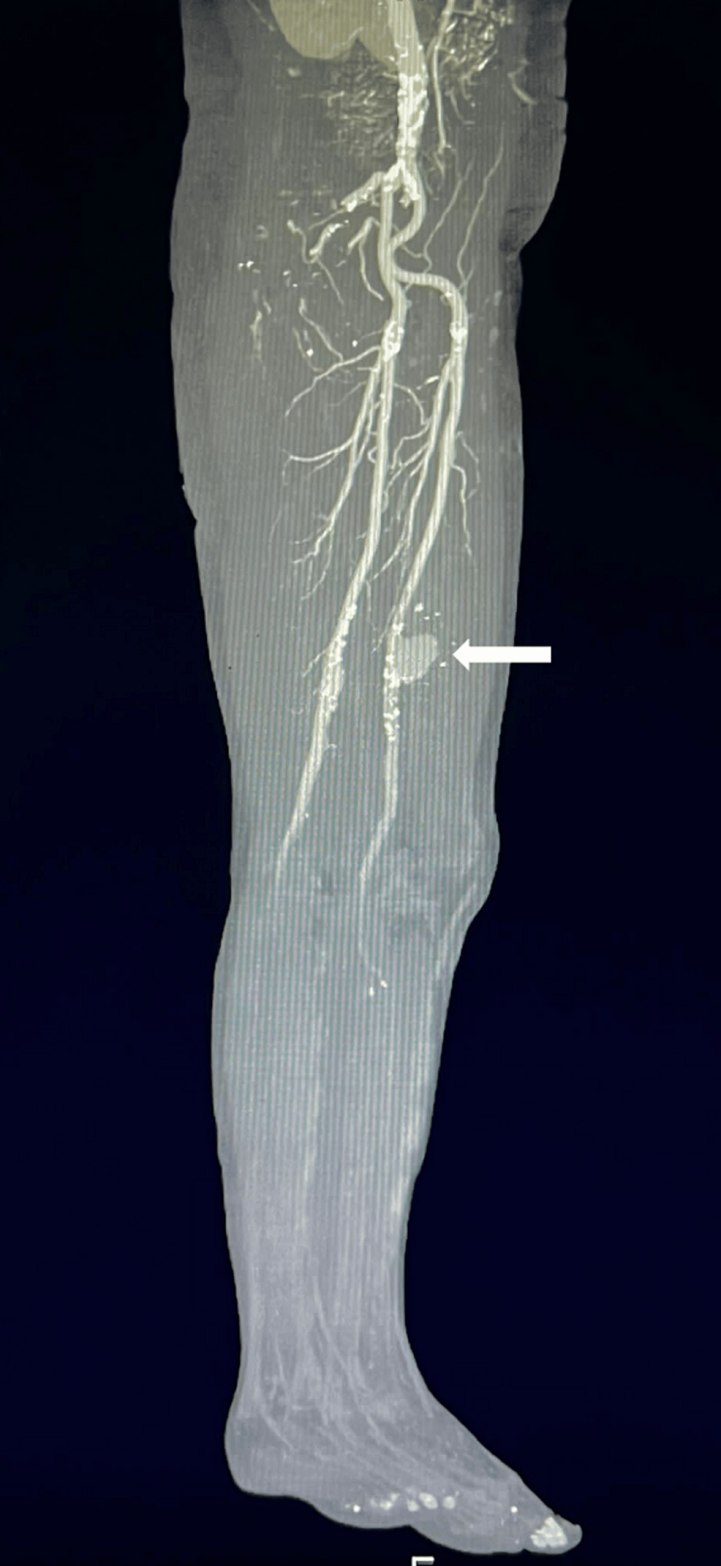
CTA revealing the left-sided saccular superficial femoral artery aneurysm protruding anteriorly. CTA: Computed tomography angiography
